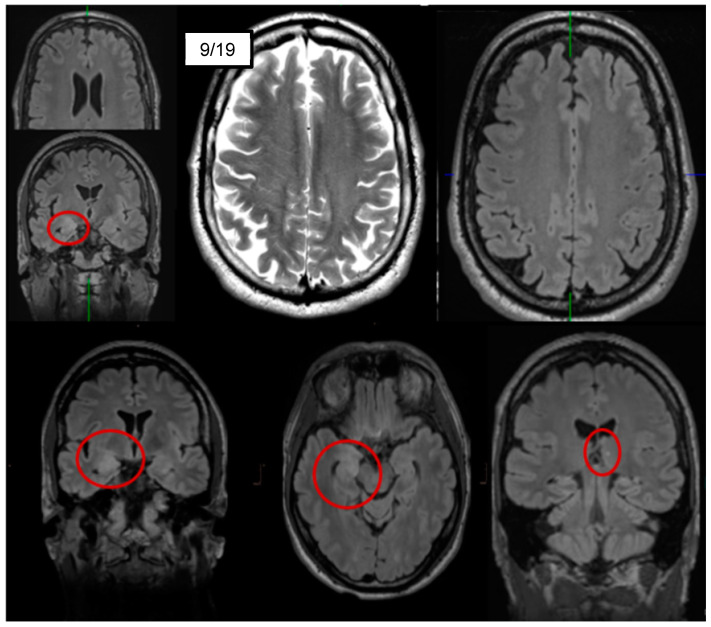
Magnetic resonance imaging showing fluid-attenuated inversion recovery (FLAIR) hyperintensities right-mesio-temporally and on the right side of the corpus amygdaloideum, as well as a small, possibly microangiopathic, lesion in the left thalamus (bottom row). In addition, a slight grey–white matter blurring was observed (top center and right; cf. [8]).
